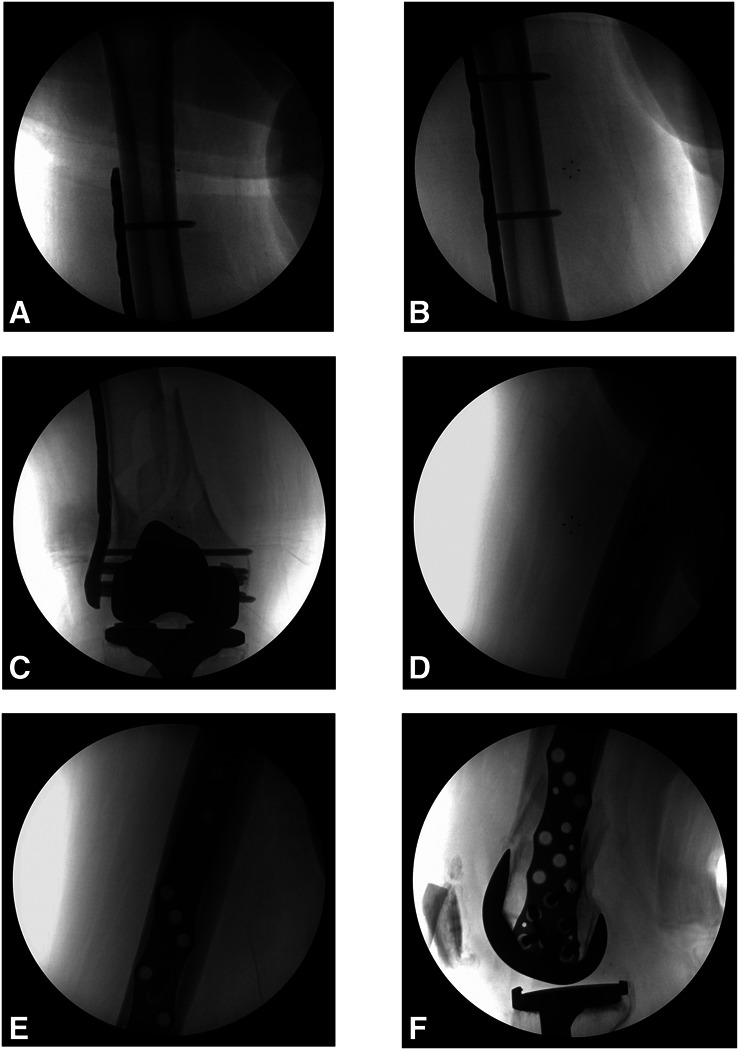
A-C, AP and (D-F) lateral intraoperative radiographs of the patient's right femur at the time of open reduction and internal fixation.
